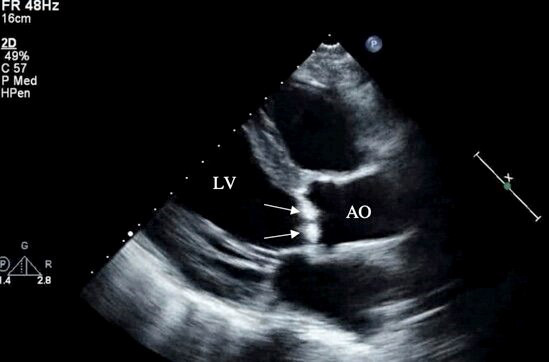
Transthoracic echocardiography (parasternal long axis view) of a
